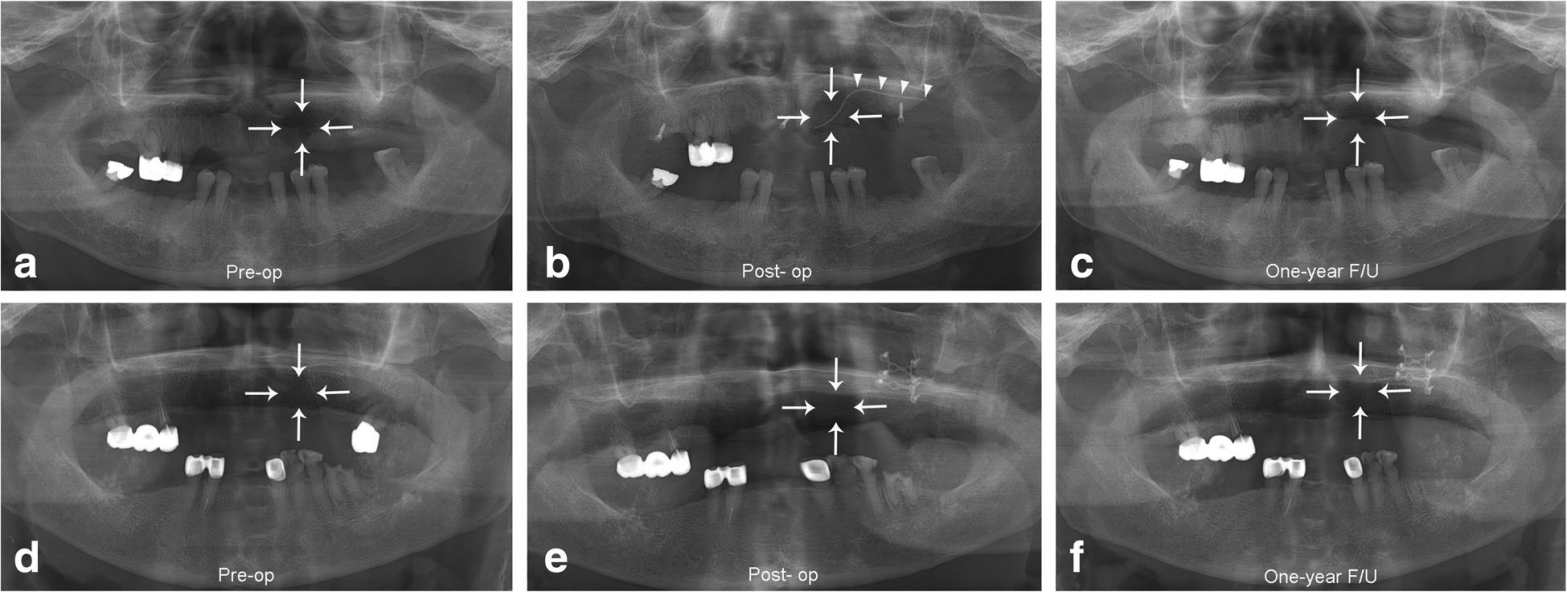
A panoramic radiograph comparing suppurative type OM with and without drainage. Pre-operative radiograph showing a suppurative OM lesion in the left posterior maxilla region (marked with arrows) in patient No. 30 (a). Immediate post-operative view following saucerization and drain insertion for decompression (marked with arrowheads) (b). A one-year follow-up radiograph showing bony healing (c). Pre-operative panoramic radiograph showing sclerotic OM in the left posterior maxilla region in patient No. 23 (d). Immediate post-operative view without drain (e). One-year follow-up view (f)
